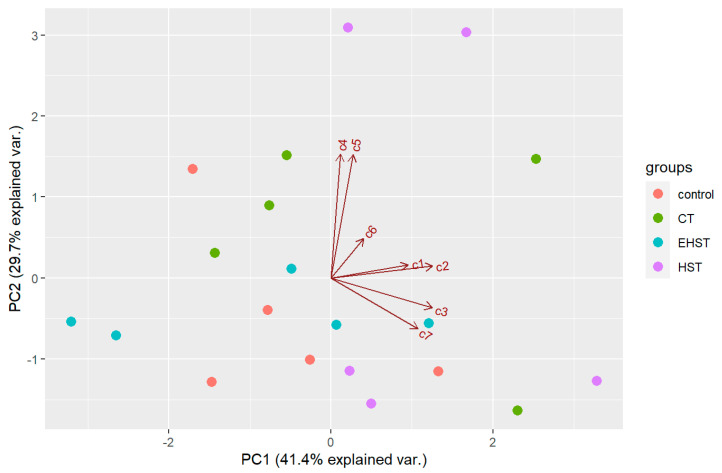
Biplot space of the PCA of the samples from the heat stress experiment. Control = natural habitat samples (Deception Island), CT = control temperature, HST = heat stress temperature, EHST = extreme heat stress temperature. c1–c7 = compounds 1–7 analysed.
